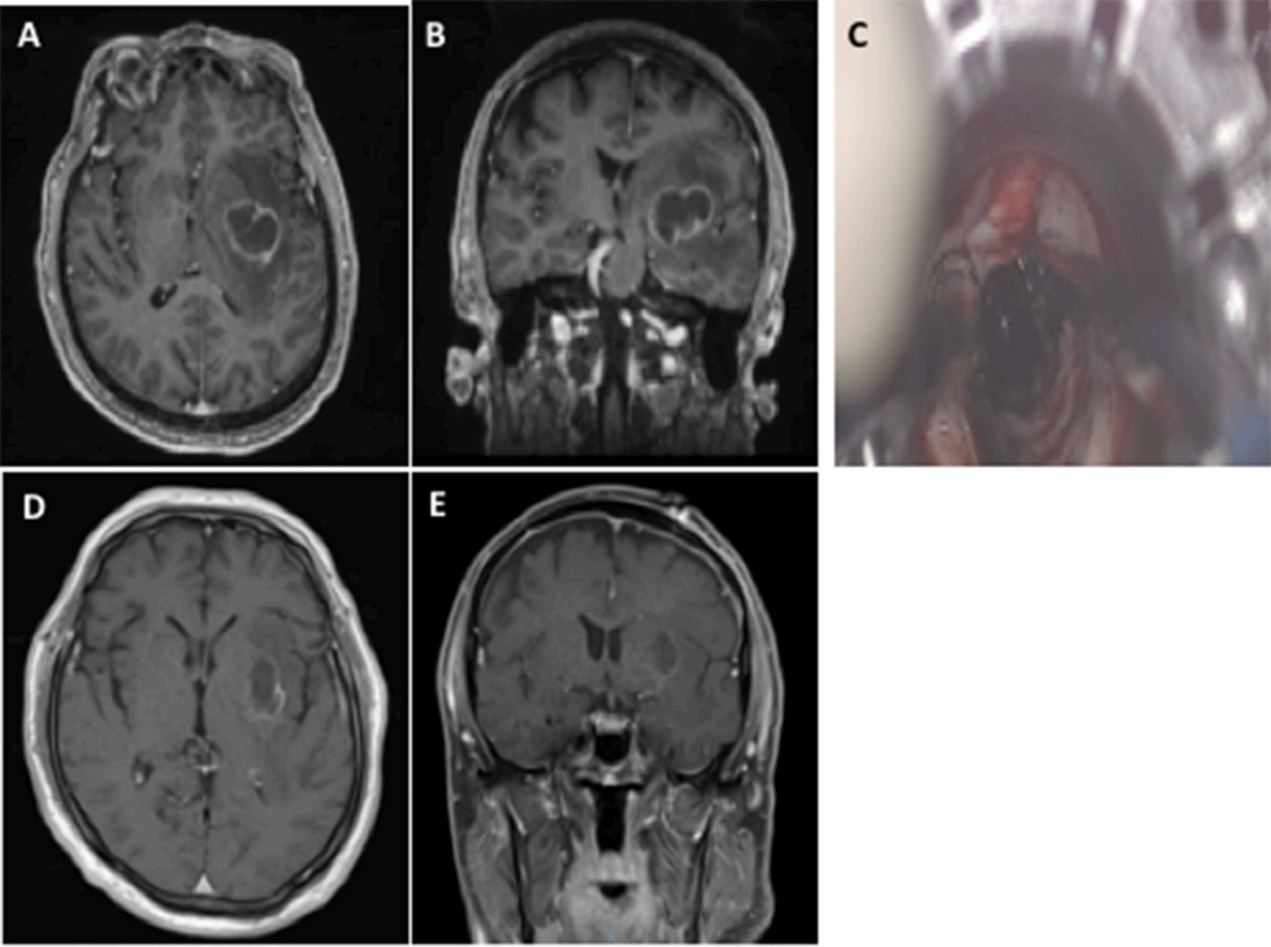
The use of channel-based retractor of a left basal ganglia non-small cell lung cancer brain metastasis. Preoperative axial (A) and coronal (B) MRI with contrast demonstrating a deep-seated left basal ganglia brain metastasis. The use of a channel-based retractor to access the lesion (C). Postoperative axial (D) and coronal (E) MRI with contrast demonstrating gross total resection and no superficial cortical and white matter changes.
